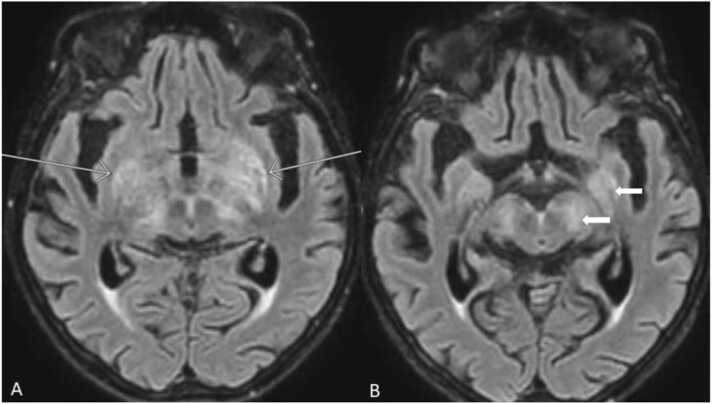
MRI FLAIR with bilateral hyperintensities in A: basal gangliae and thalami (arrows), B: medial temporal lobes and mesencephalon (wide arrows). There was no abnormal contrast enhancement including meninges and no areas of restricted diffusion (not shown). These changes completely resolved on a follow-up MRI (not shown).
